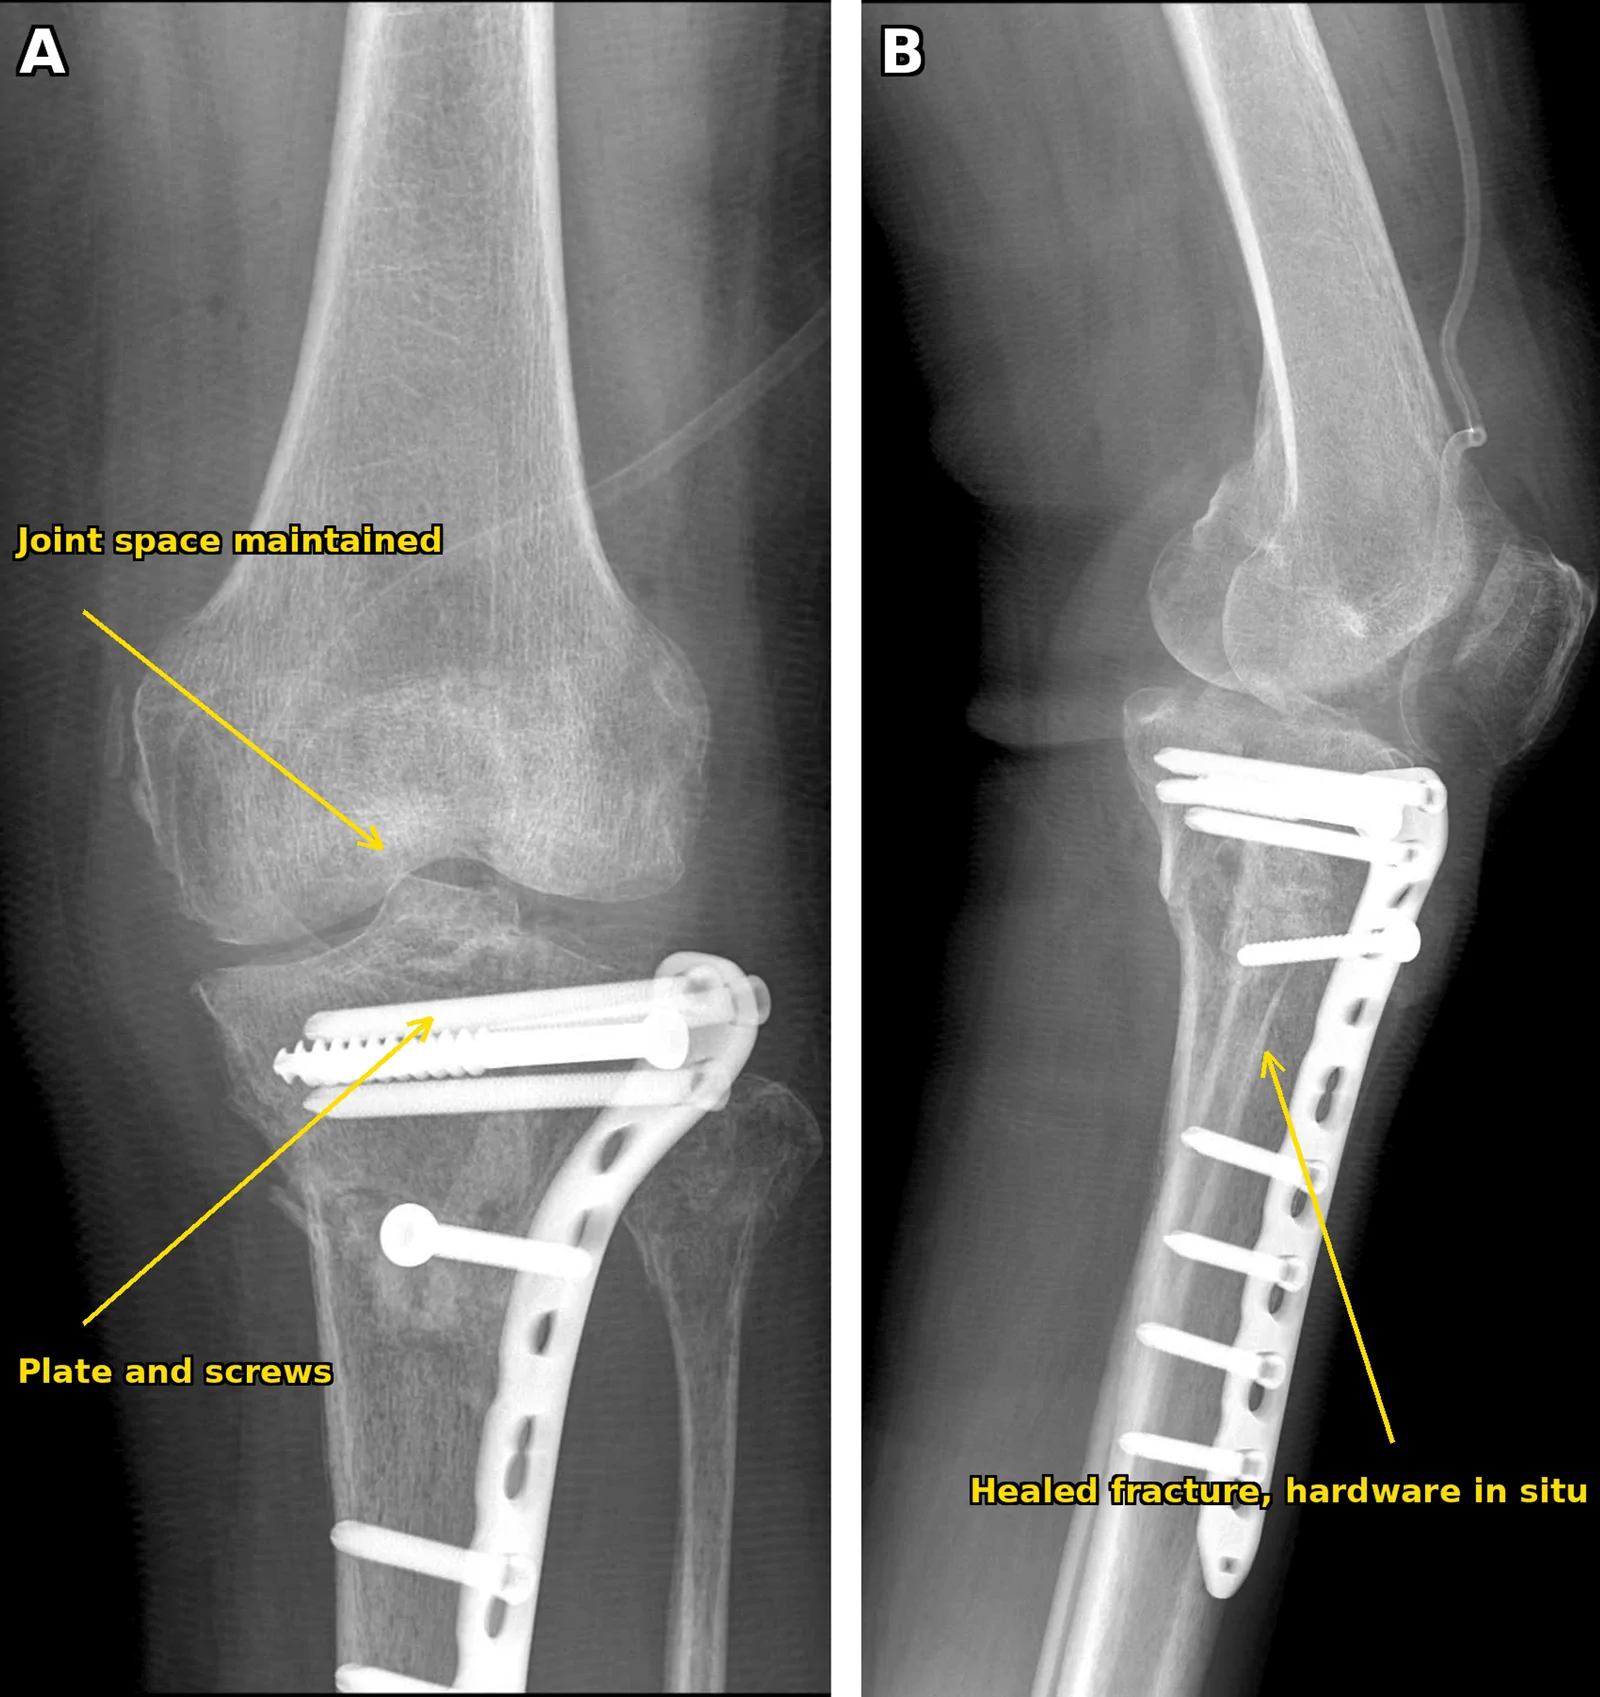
Preoperative radiographs of the left knee after index fixation. (A) Anteroposterior view showing the lateral buttress plate and screws in situ (arrow) with a maintained tibiofemoral joint space (arrow). (B) Lateral view showing the healed proximal tibial fracture with hardware in situ (arrow), without failure, loosening, or malposition. Diffuse periarticular osteopenia of the distal femur and proximal tibia is evident on both views.
